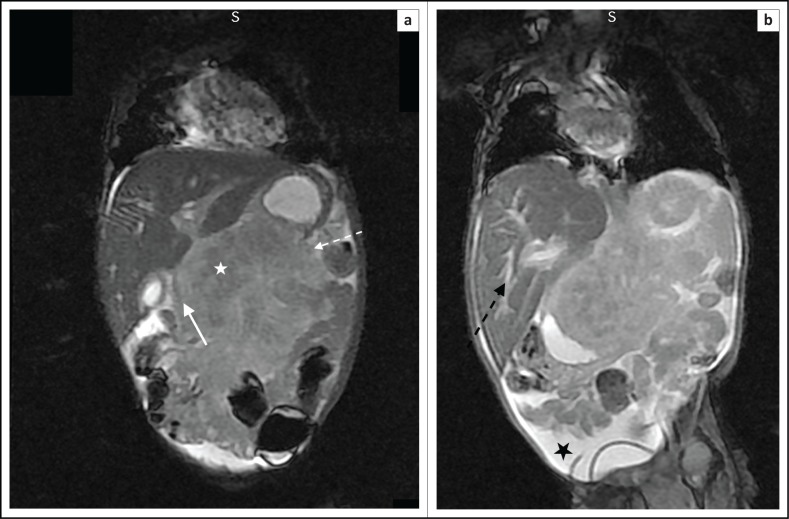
Coronal T2-weighted (T2W) magnetic resonance images: (a) Pancreatic mass (white star) with ill-defined margins and a lobulated contour (broken white arrow). Evidence of peripancreatic extension to the porta hepatis. This lesion is locally invasive, as demonstrated by the inseparability from the D2 segment of the duodenum (solid white arrow); (b) Associated findings of dilated intrahepatic bile ducts (broken black arrow) and ascites (black star).
